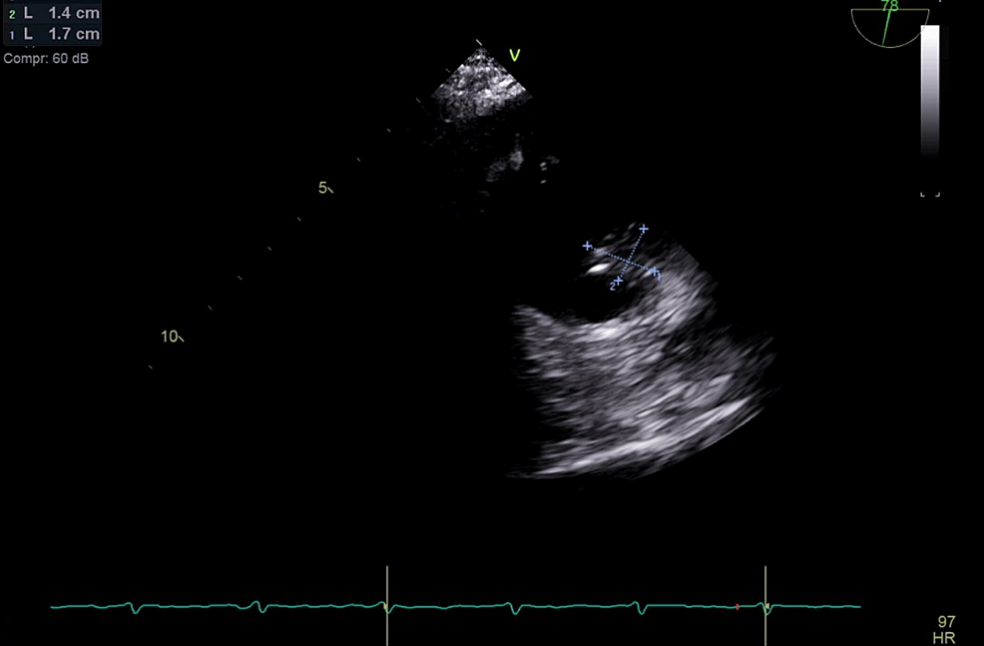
Transesophageal echocardiography demonstrates a vegetation measuring 1.4 × 1.7 cm in the right atrium
